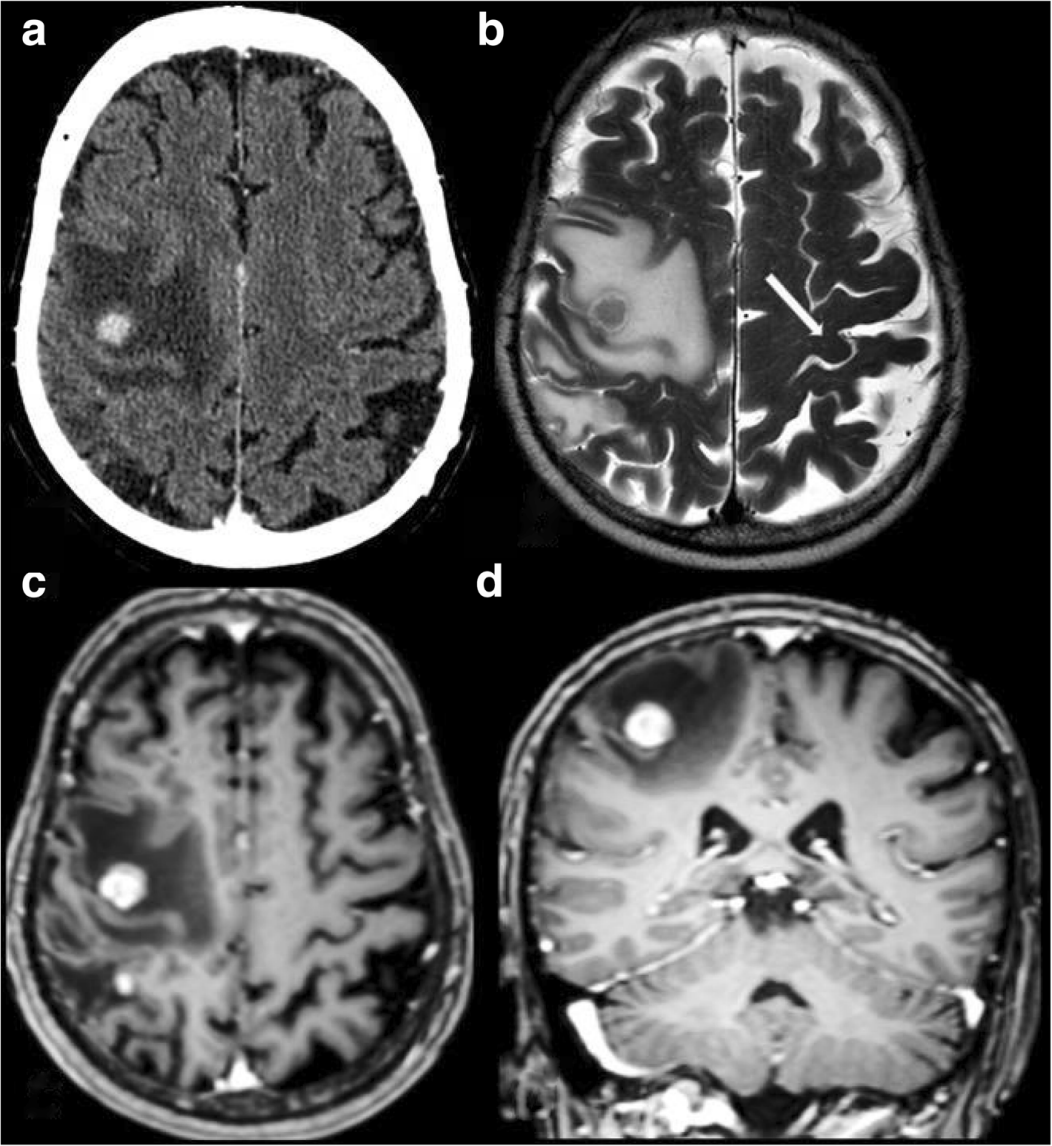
Contrast-enhanced axial CT (a), T2W axial MR (b), contrast-enhanced T1W axial (c) and coronal (d) MR. Intensive contrast enhancement (11 mm) in the right precentral gyrus, corresponding to the ‘hand knob’s area, with large perifocal edema. The left ‘hand knob’ is normal (arrow). Four other smaller enhancing metastases in the brain (not shown)
